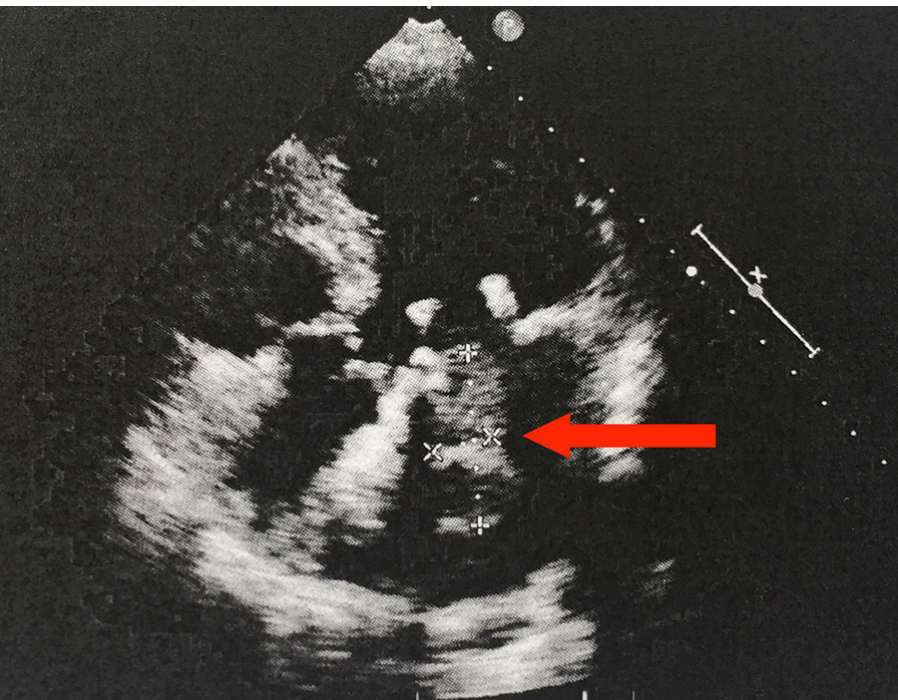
Echocardiogram, four-chamber view The 70-year-old female patient developed a space-occupying lesion in the left atrium. The echocardiogram shows a 4.05×1.39 cm echogenic mass attached to the mitral valve. The arrow indicates the mass.
